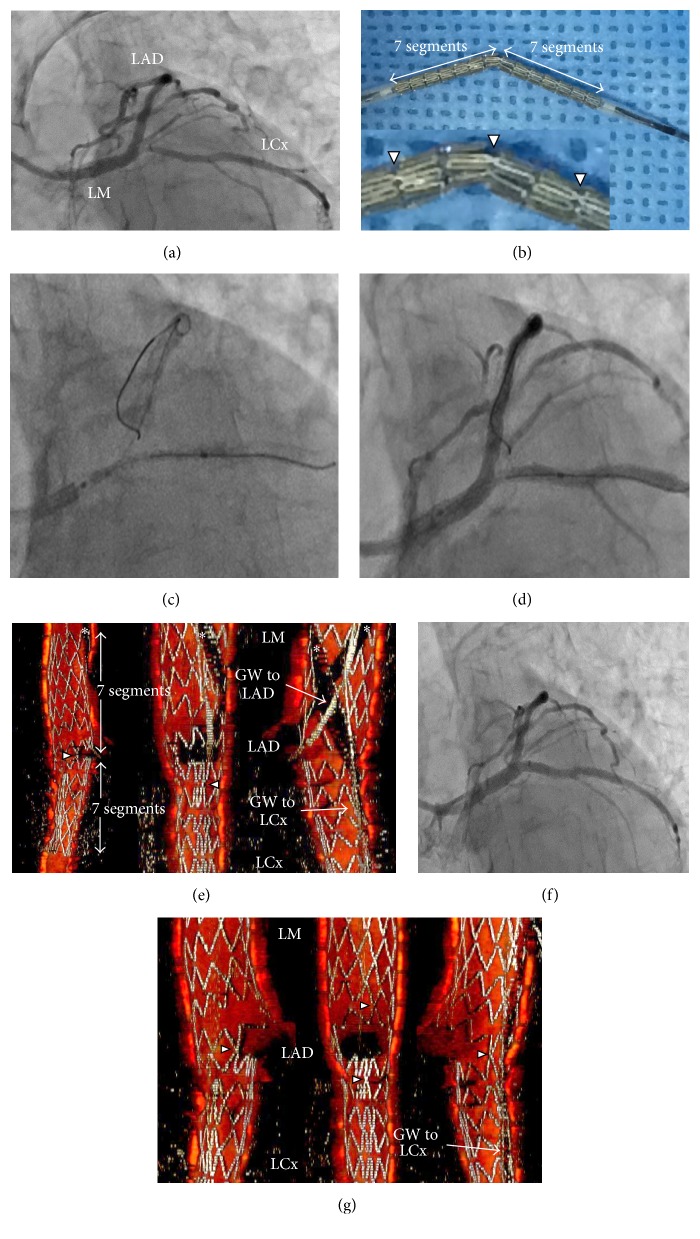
First clinical case treated with the bent stent technique. (a) Baseline coronary angiography (CAG). (b) 3.5 × 18 mm two-link Biolimus-eluting stent is bent at the center articulation with laterally positioned links. Stenting position is confirmed by X-ray fluorography (c) and CAG (d). (e) Instant stent-accentuated three-dimensional optical coherence tomography (iSA3D-OCT) shows free carina type and distal cell rewiring. After kissing balloon dilation is performed, final CAG (f) and iSA3D-OCT (g) show a good result. LM: left main coronary artery; LAD: left anterior descending artery; LCx: left circumflex artery; GW: guidewire. Arrowheads and asterisks indicate links and guidewire shadow artifacts, respectively.
